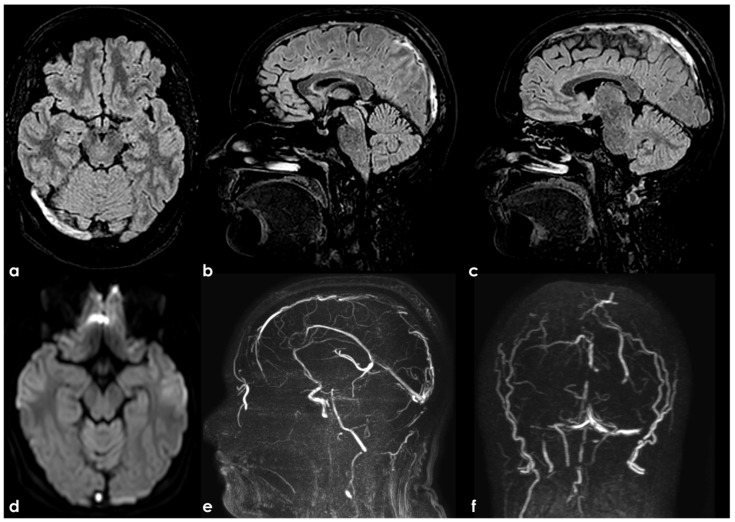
Brain MRI and PC-MRV in a woman with subacute CVT involving the SSS and TS on both sides, sparing the torcular. Axial and sagittal FLAIR (panels (a) and (b,c), respectively) shows the spontaneous hyperintensity of the right TS and SSS. The clot in the right TS shows a focal hyperintensity in diffusion-weighted imaging (DWI) (panel (d)). PC-MRV shows multiple irregular flow defects in the SSS and TS on both sides (panels e,f).
